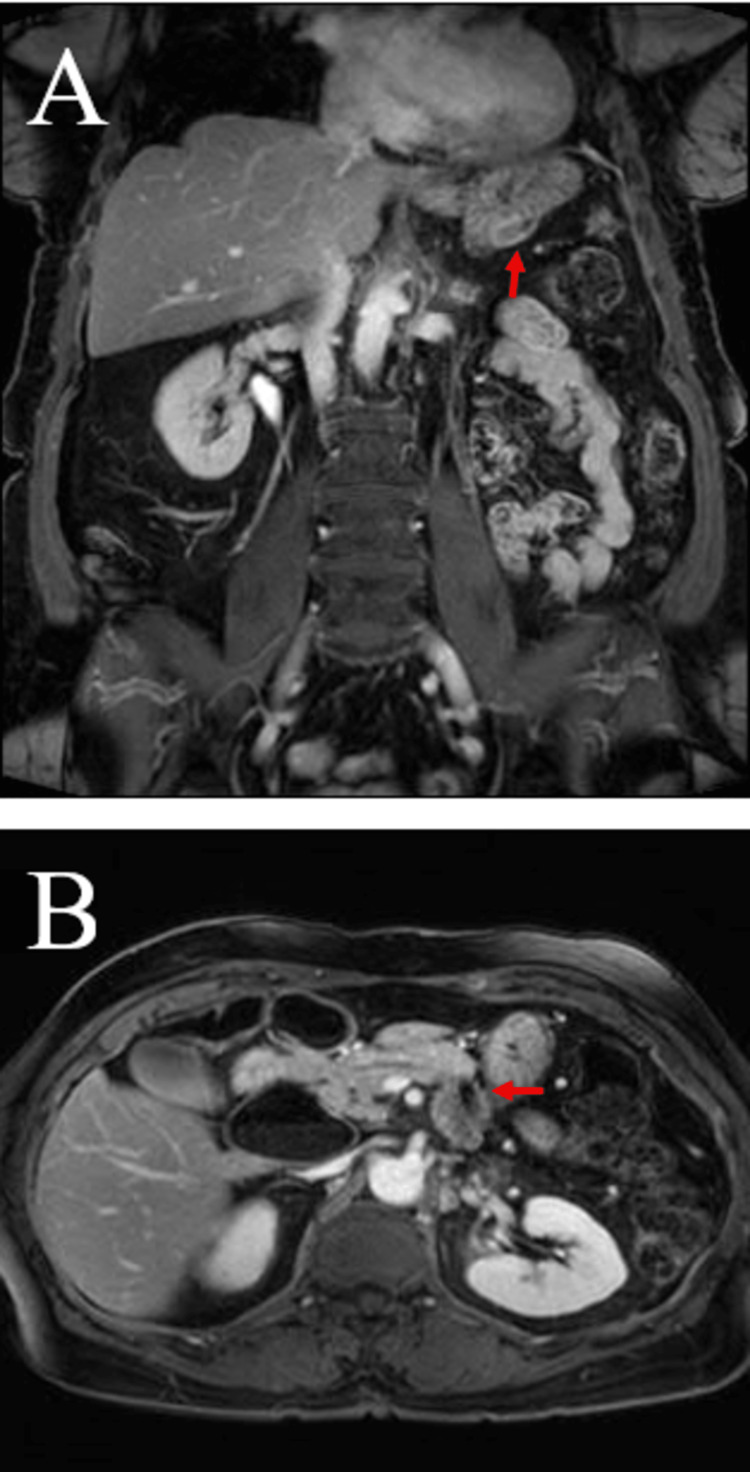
MRI of the abdomen/pelvis showing peritoneal lesions. A) 1.9 cm implant along the undersurface of the gastric body. B) 3.2 × 1.5 cm lesion along the pancreatectomy margin (previously 2.4 × 1.2 cm).
